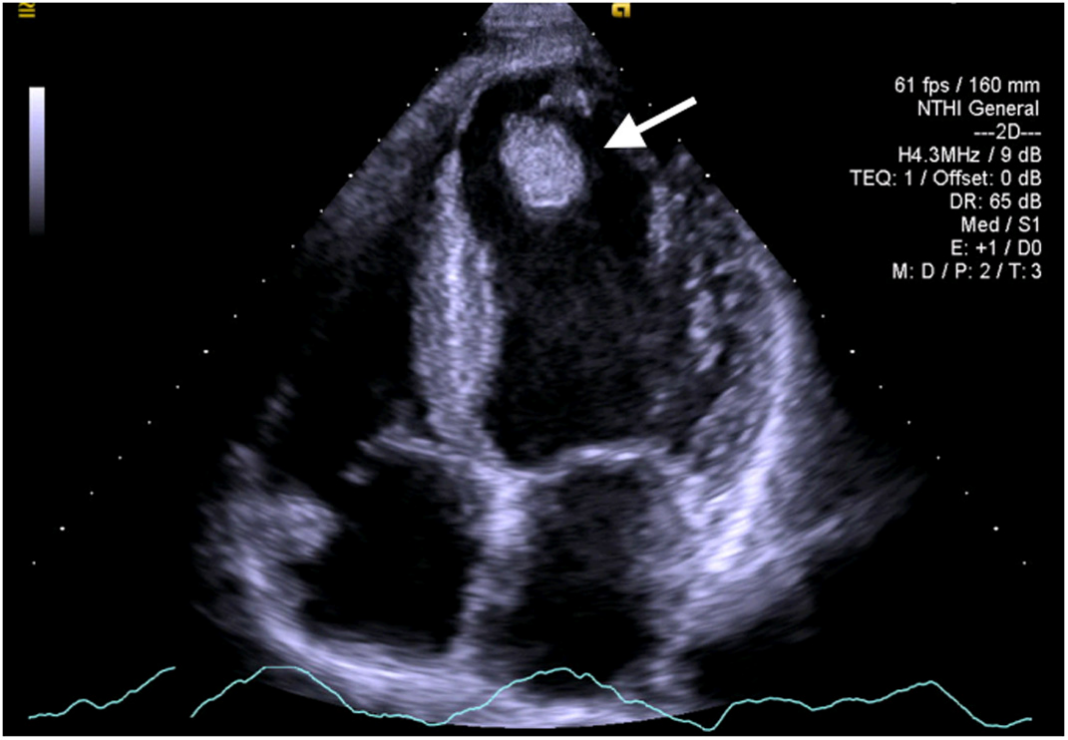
Transthoracic Echocardiogram on Admission A mobile thrombus measuring 21 × 15 mm is visible in the left ventricular apex (arrow).
